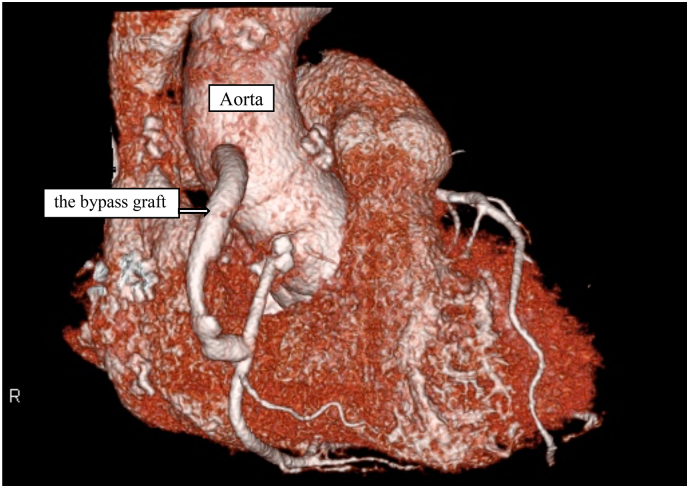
Postoperative computed tomography for the initial surgical procedure The aneurysm within the sinus of Valsalva appears thrombosed and devoid of any discernible contrast enhancement. Furthermore, the bypass graft originating from the ascending aorta and directed toward the second right coronary artery remains open and unobstructed.
